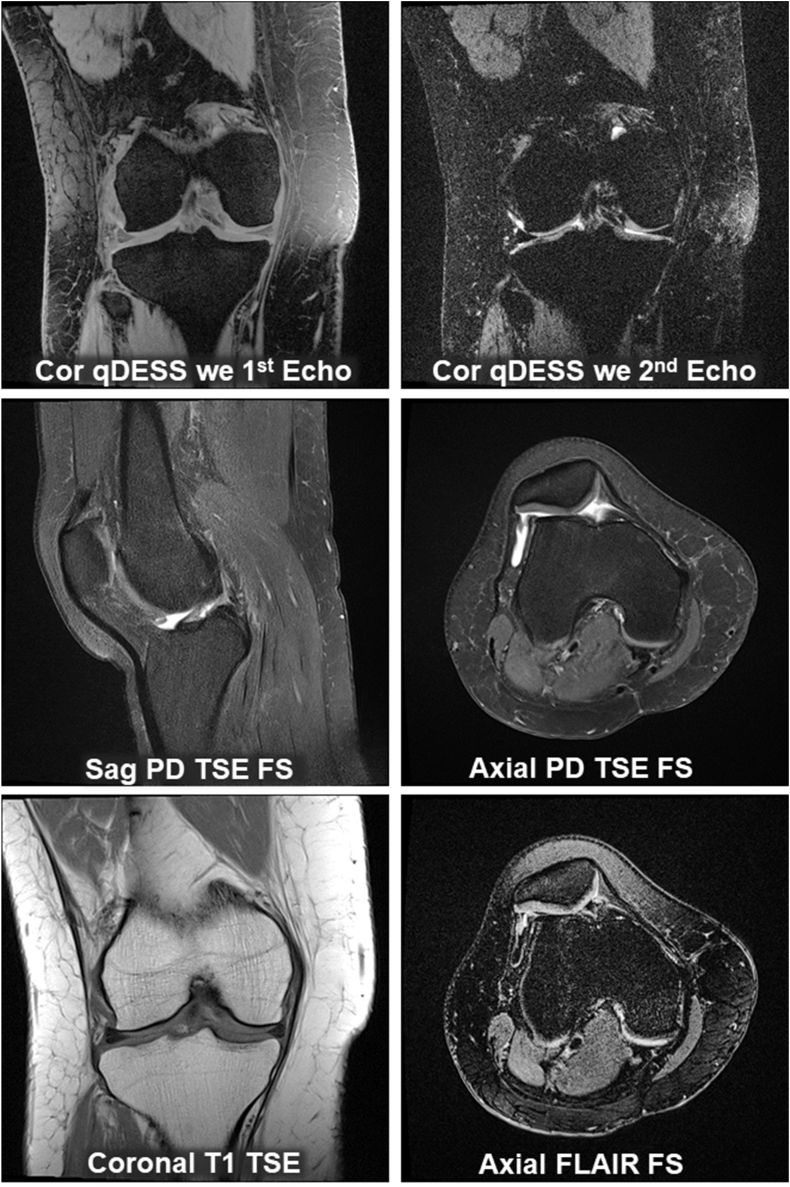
Example images of the proposed sample MRI acquisition protocol for clinical trials. These encompass the 1st and 2nd echo of the qDESS MRI sequence with water excitation (we). The 1st echo displays a mixed T1/T2 contrast, whereas the second one exhibits T2 and diffusion weighted imaging contrast. The “clinical” sequences in three orientations include a sagittal (sag) PD weighted TSE sequence with fat-saturation (FS) as well as an axially orientated PD TSE FS; the coronal (Cor) TSE sequence is acquired with T1 weighting (T1) and without FS. Finally, an axial FLAIR is obtained with FS. The MRIs were obtained on a 3T Siemens VIDA scanner (Software Version Numaris VA 50).
